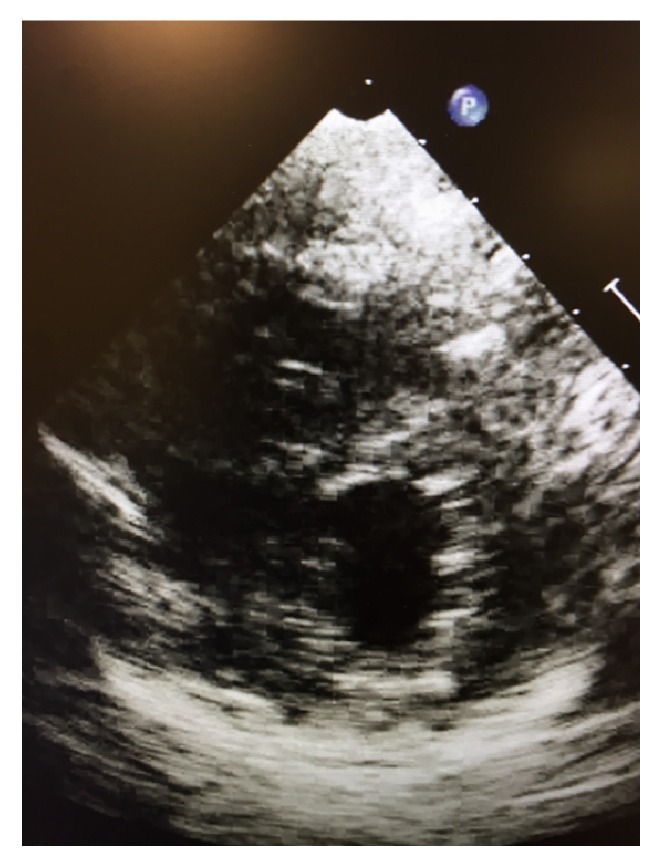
Echocardiogram parasternal short axis showing left ventricular chamber back to normal size and function.
